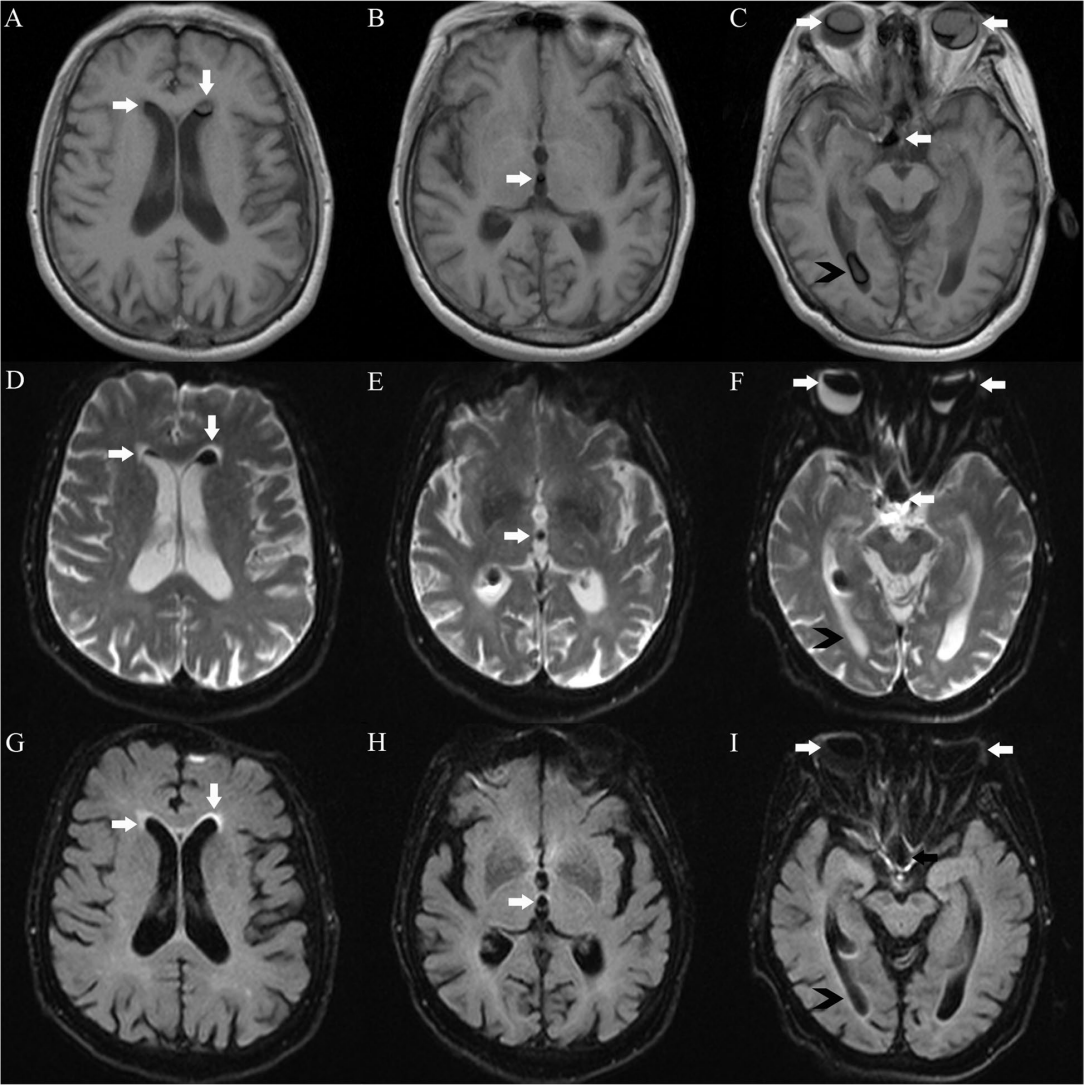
Brain MRI revealed abnormal signals in the bilateral eyeballs, bilateral lateral ventricle anterior horns, right lateral ventricle posterior horn, third ventricle, and right suprasellar cistern, showing hyperintensity (relative to the cerebrospinal fluid), surrounded by low-signal chemical shift artifacts, on T_1_WI (A-C); variable signals (hypo- or hyperintensity, white arrows and black arrows, respectively) on T_2_WI (D-F); hypointensity on FLAIR (G-I). The silicone particles in the anterior horn of the right lateral ventricle on MRI (2A and 2D, white arrows) was smaller than that on CT (1A, white arrow) and mostly shifted to the posterior horn of the right lateral ventricle (2C, 2F, and 2I, black arrows)
